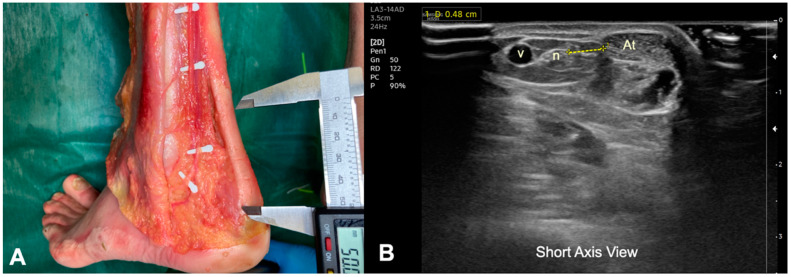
(A) Anatomical scheme of the course of the sural nerve and its relationship with the Achilles tendon on the lateral aspect of the ankle (white pins). Measurement in cadaver at 5 cm from the insertion in the calcaneus. (B) Ultrasound image (short axis view) of the relationship and measurement of the sural nerve with the lateral border of the Achilles tendon in a healthy volunteer. (At)—Achilles Tendon, (n)—Sural nerve, (v)—Lesser saphenous vein.
